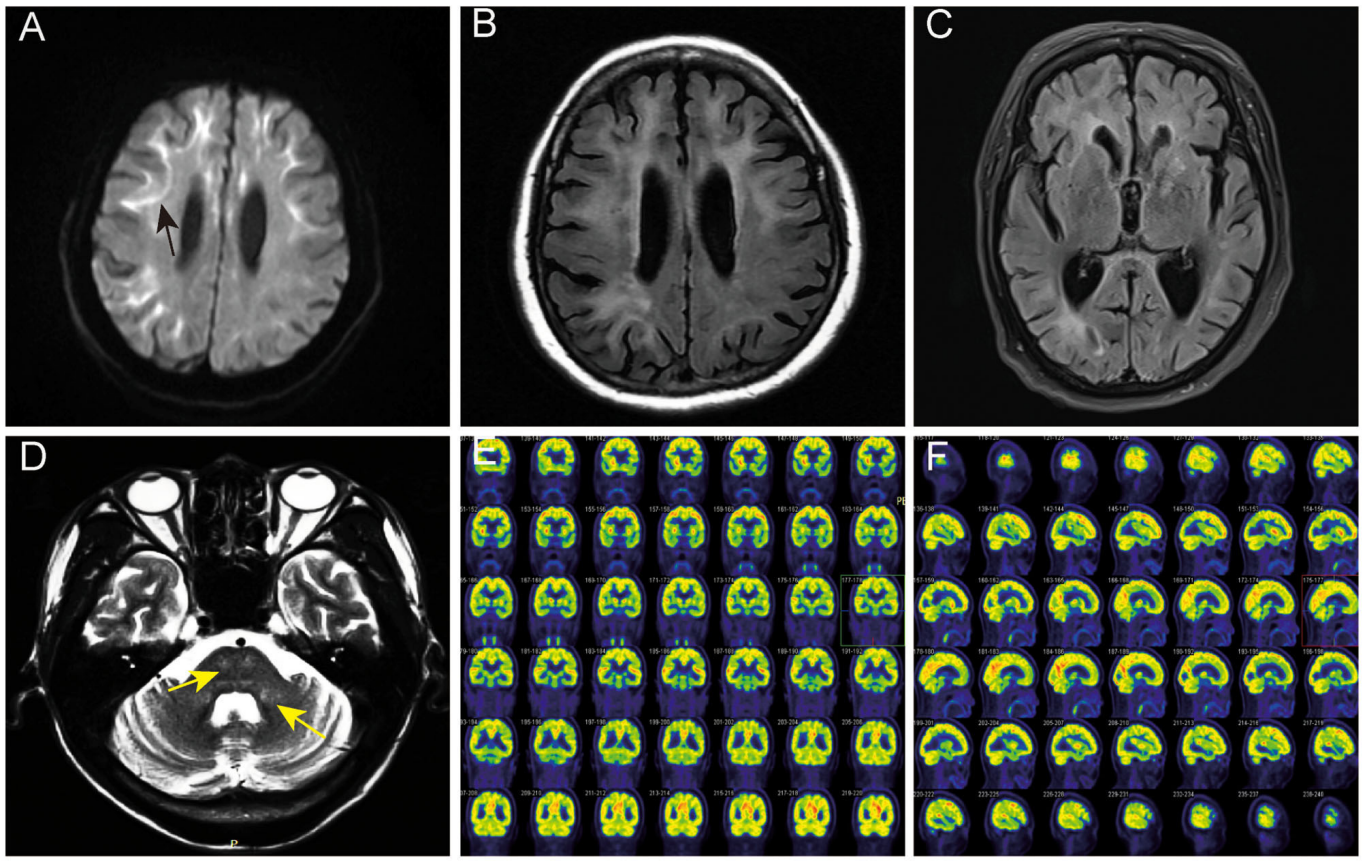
Head MRI and PET-CT findings of NIID cases. (A) The linear high signals on DWI of subject 2 in the corticomedullary junction (black arrow). (B) Bilateral paraventricular white matter hyperintensity on T2 FLAIR of subject 2. (C) Ventricular dilation on T2 of subject 2. (D) Hyperintensity areas in the cerebral pontine and the middle cerebellar peduncles on T2 FLAIR (yellow arrow). (E,F) The^18^F-FDG PET-CT of subject 2 showed decreased metabolism in the left frontal lobe and right parietal lobe.
